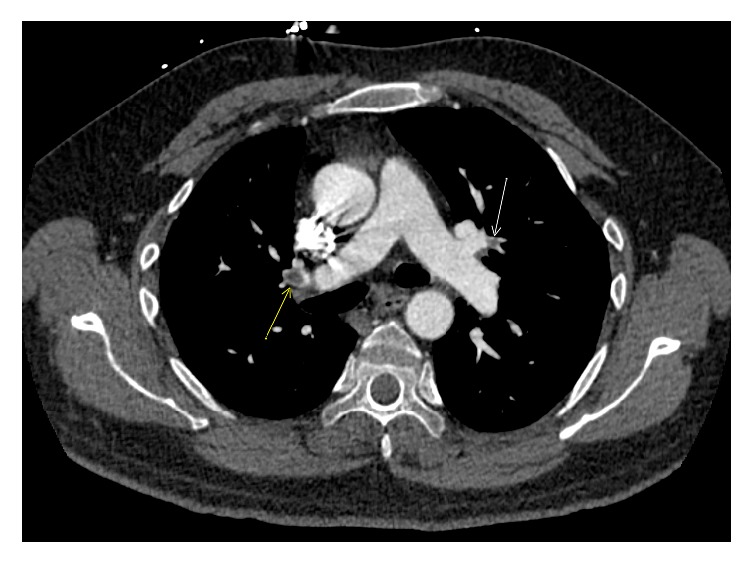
Axial CT image of the chest at the level of the main pulmonary artery bifurcation. Arrows denote two of the multiple pulmonary artery emboli (filling defects) which were observed bilaterally in this patient within both lobar and segmental pulmonary arteries.
